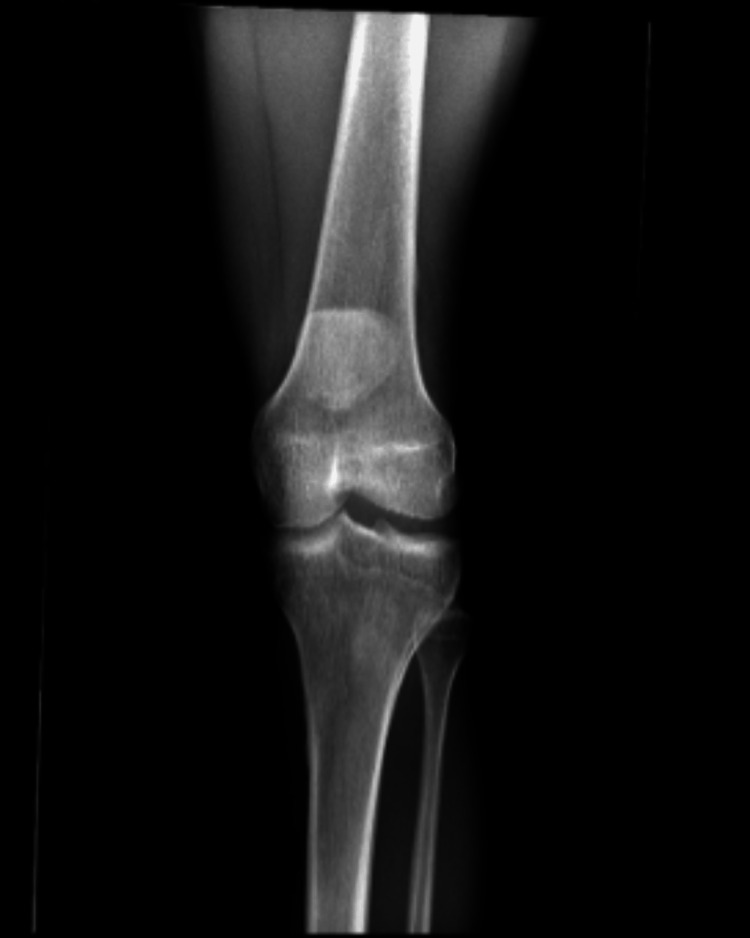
X-ray (anteroposterior view) post-reduction
